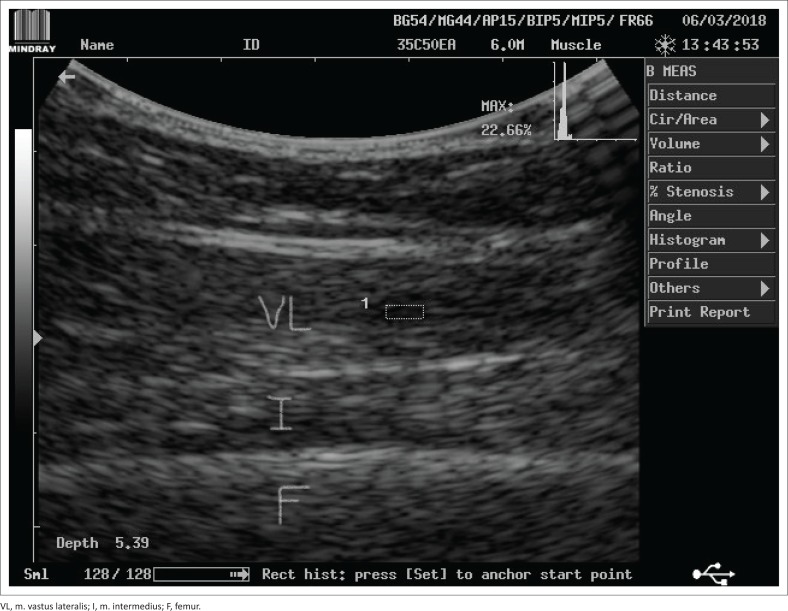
Example of measurement of echo intensity of the vastus lateralis muscle of a healthy individual using on-screen callipers of the ultrasound diagnostic imaging system.
